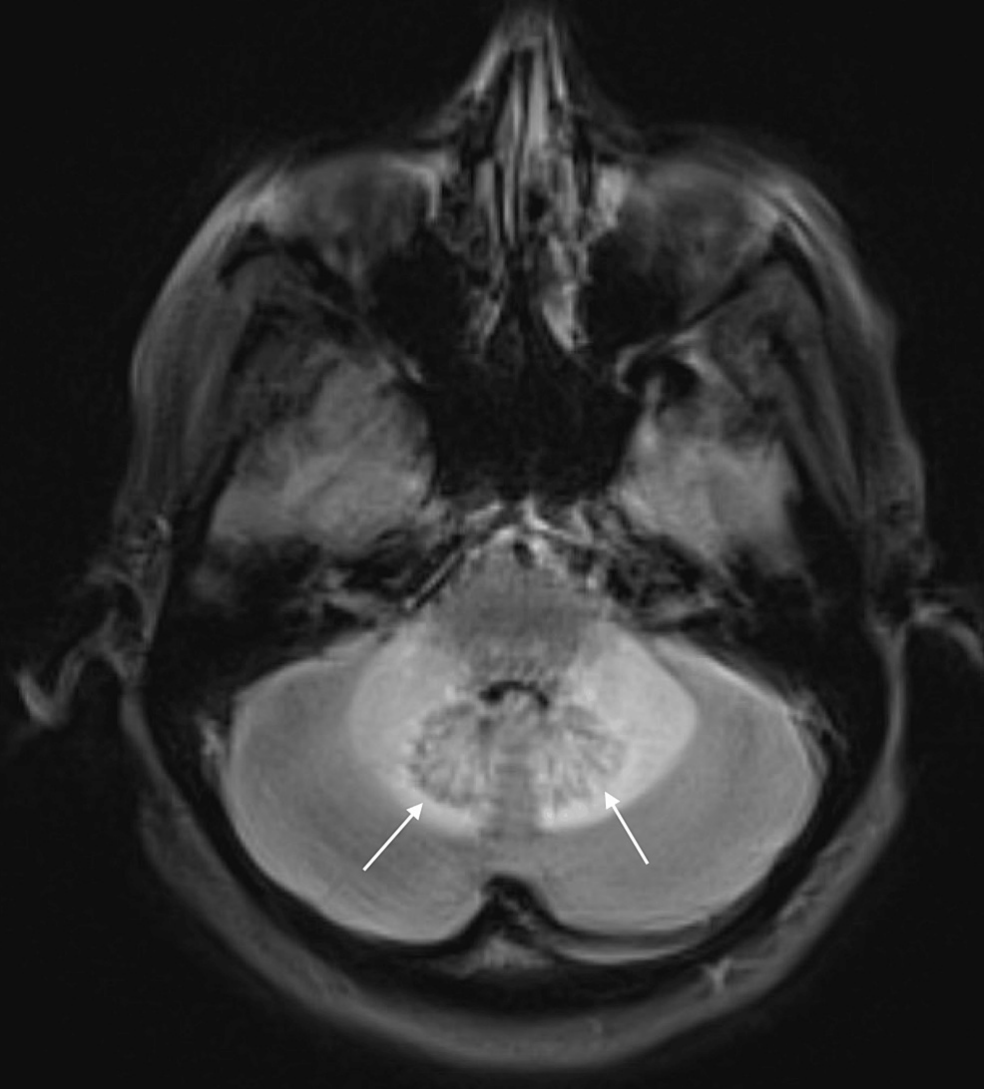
T2 FLAIR images demonstrate symmetrical involvement of the cerebellar white matter and middle cerebellar peduncles in a “butterfly wing” pattern (Arrows). FLAIR: fluid-attenuated inversion recovery
